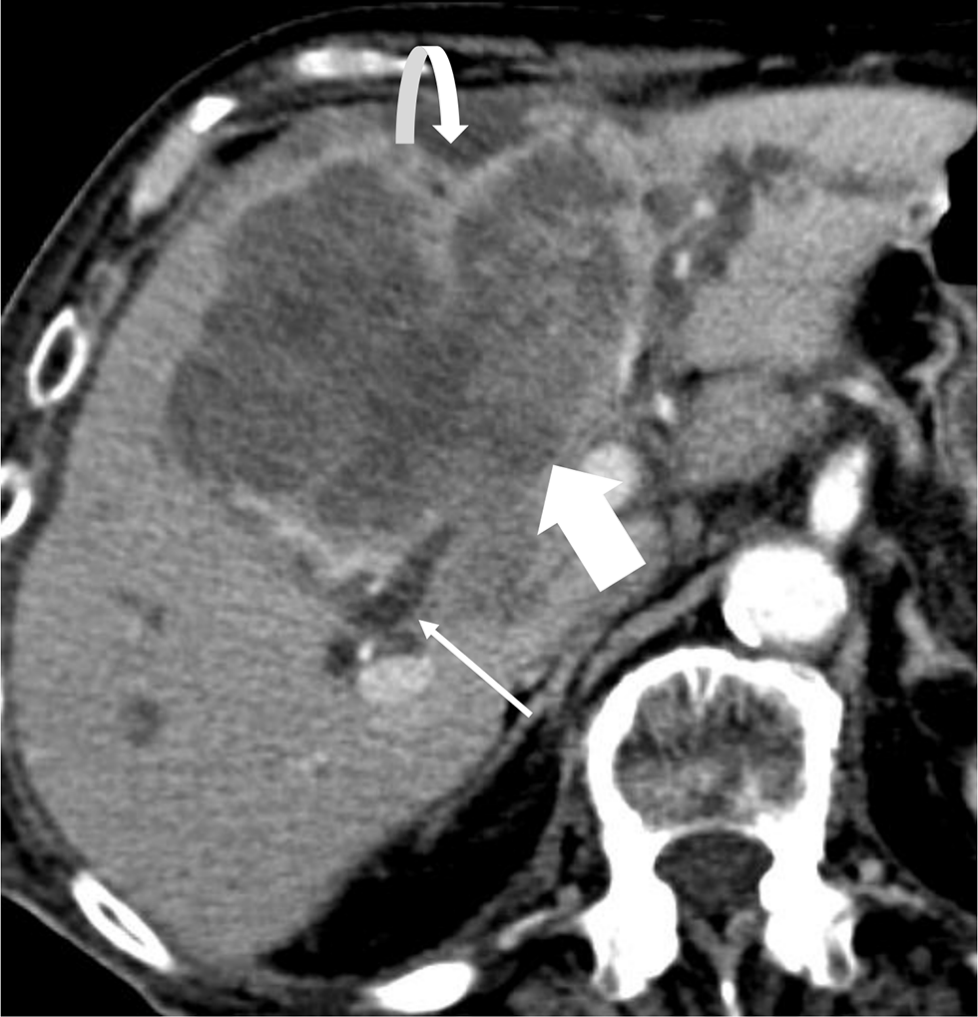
Hepatic Capsular Retraction. Axial contrast-enhanced CT demonstrates a large central mass forming CCA (block arrow) with hepatic capsular retraction (curved arrow) along the capsule of the liver. Also noted are dilated upstream bile ducts (thin arrow)
